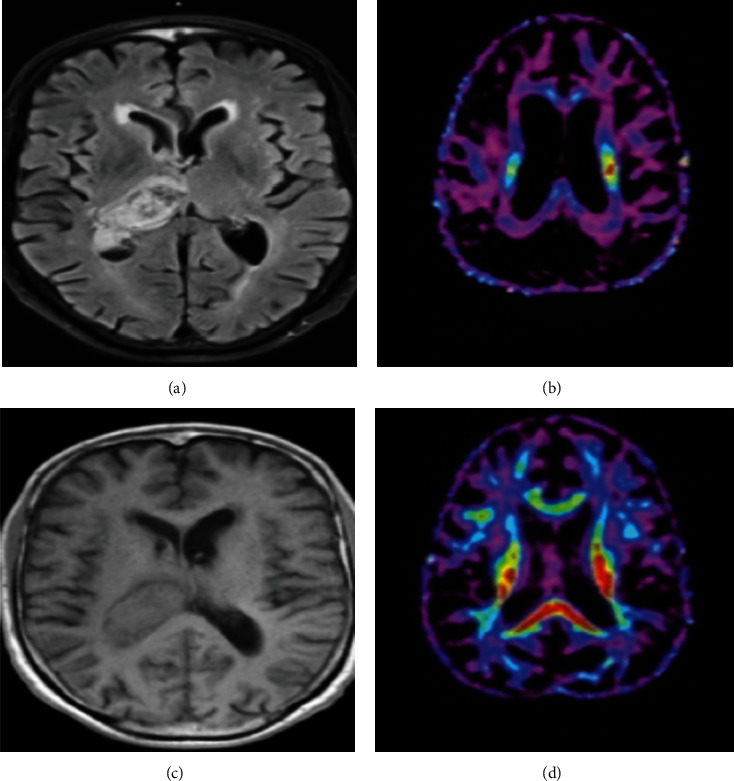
(a) Preoperative MRI images of intracerebral hemorrhage in the right brain area. The internal capsule and the anterior horn of the lateral ventricle were compressed to a certain extent. (b) DTI images of the bilateral corticospinal tracts before the operation. The conduction tracts were damaged, causing relaxation and partial disconnection. (c) One month after the operation, the hematoma was partially absorbed, and the pressure on the frontal angle of the lateral ventricle was weakened. (d) One month after the operation, the morphology of the corticospinal conduction tract was improved, and the broken conduction tract was partially recovered.
